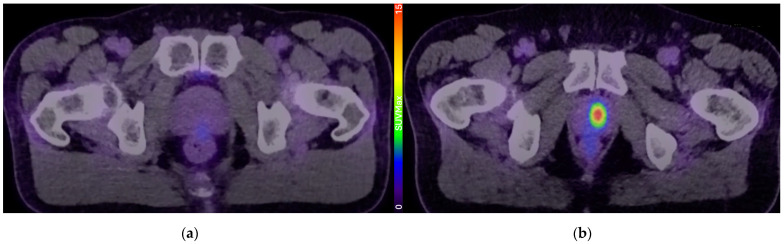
Example PSMA PET/CT scans demonstrating intra-prostatic uptake of radiolabelled PSMA tracer. (a) PSMA scan demonstrating low intraprostatic uptake—PRIMARY score of 1; (b) PSMA scan demonstrating a focal area of high tracer uptake—PRIMARY score of 5.
